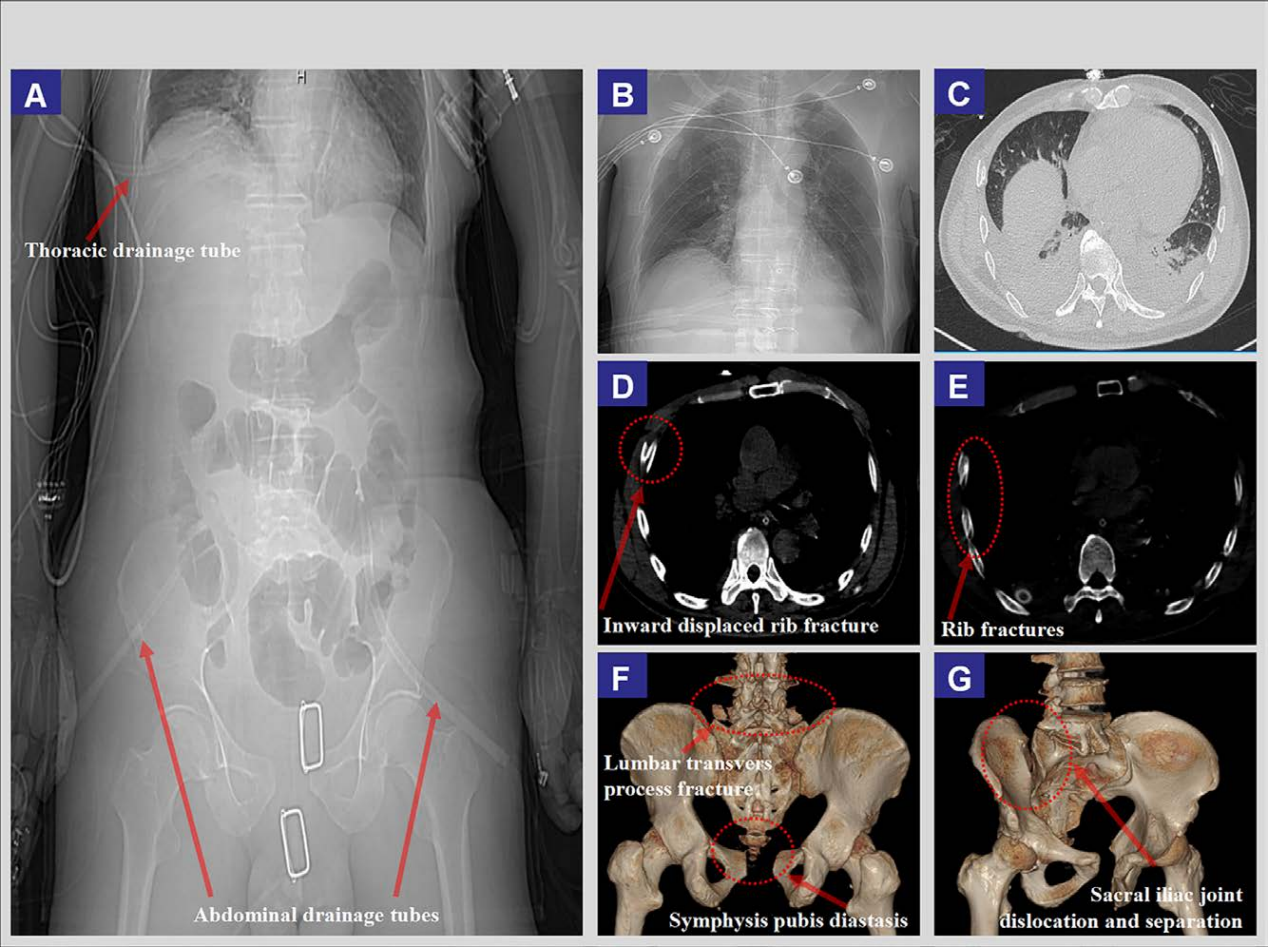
Emergency CT imaging data of the patient with multiple trauma. CT = computed tomography.
